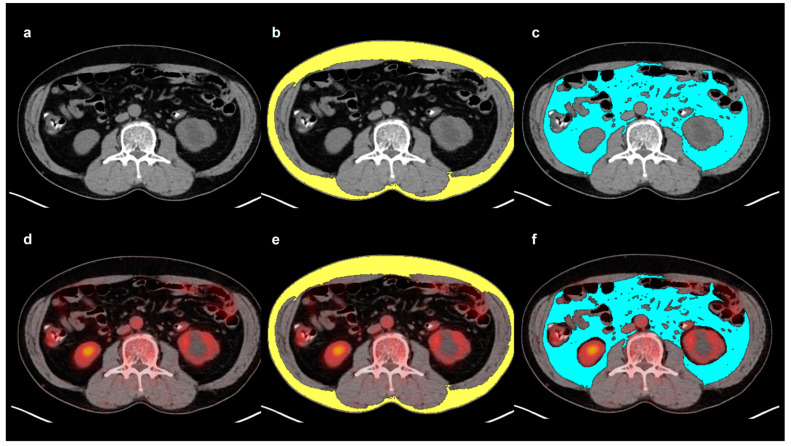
Example measurement of adipose tissue imaging parameters from FDG PET/CT images. Transaxial CT (a) and fused PET/CT (d) images at the level of the third lumbar vertebra were selected for the measurement. Areas of SAT (b) and VAT (c) were defined on CT image using HU thresholds of −190 to −30 for SAT (yellow) and −150 to −50 for VAT (blue). The area and the mean HU of both SAT and VAT were measured. Afterward, the SAT and VAT areas on CT images were exported to the corresponding fused PET/CT images (e,f). After removing the FDG activity of the urine, bowels, and vessels, the mean SUV of SAT and VAT were measured.
